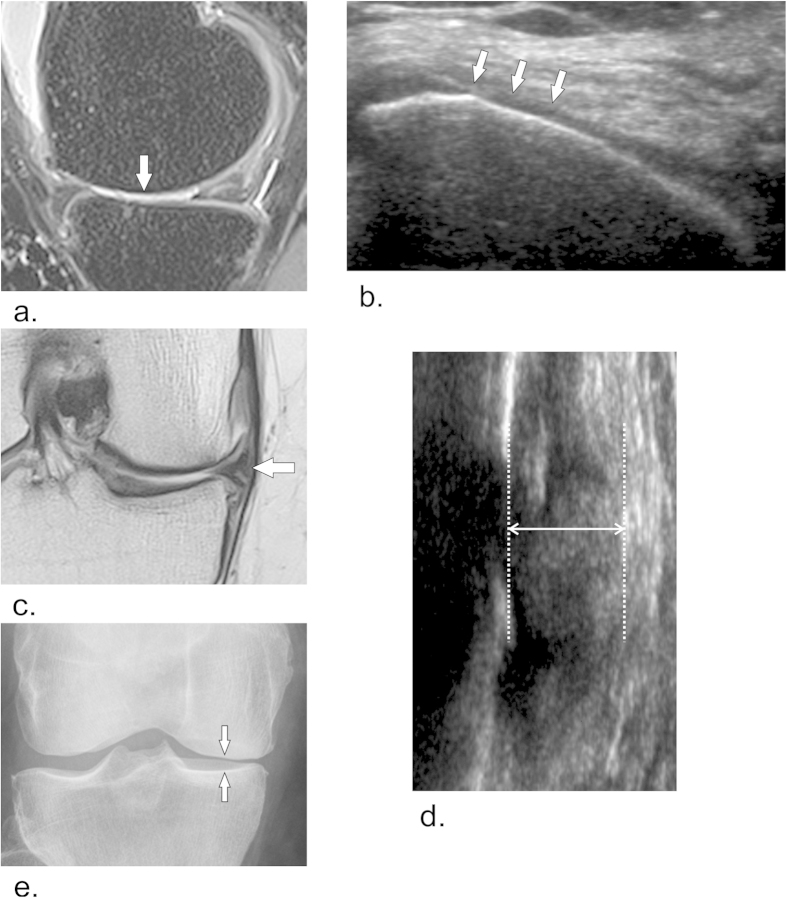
Example of structural cartilage changes and meniscal extrusion as seen in magnetic resonance images (MRI), ultrasound images and radiograph of 57-year-old symptomatic woman. Medial femoral condyle cartilage thinning indicated by white arrows in sagittal proton density weighted fat-suppressed MRI (a) and ultrasound transversal B-mode image (b). Medial meniscal extrusion can be observed in coronal proton density weighted MRI (c) (white arrow) and longitudinal B-mode ultrasound image (d) (double headed arrow). Anterior-posterior radiograph (e) demonstrates medial joint space narrowing (white arrows) as a surrogate of meniscal and cartilage structural changes.
